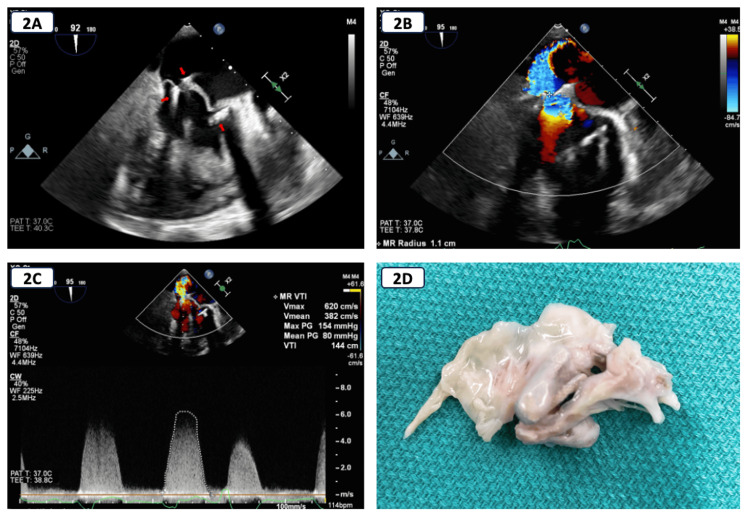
Transesophageal echocardiogram and gross pathology. A: Transesophageal echocardiogram showing mitral apparatus with three MitraClips (arrowheads). B: Transesophageal echocardiogram showing eccentric posterolaterally directed mitral regurgitation (MR) jet. C: Transesophageal echocardiogram showing MR Doppler trace. D: Resected native mitral valve with MitraClips.
